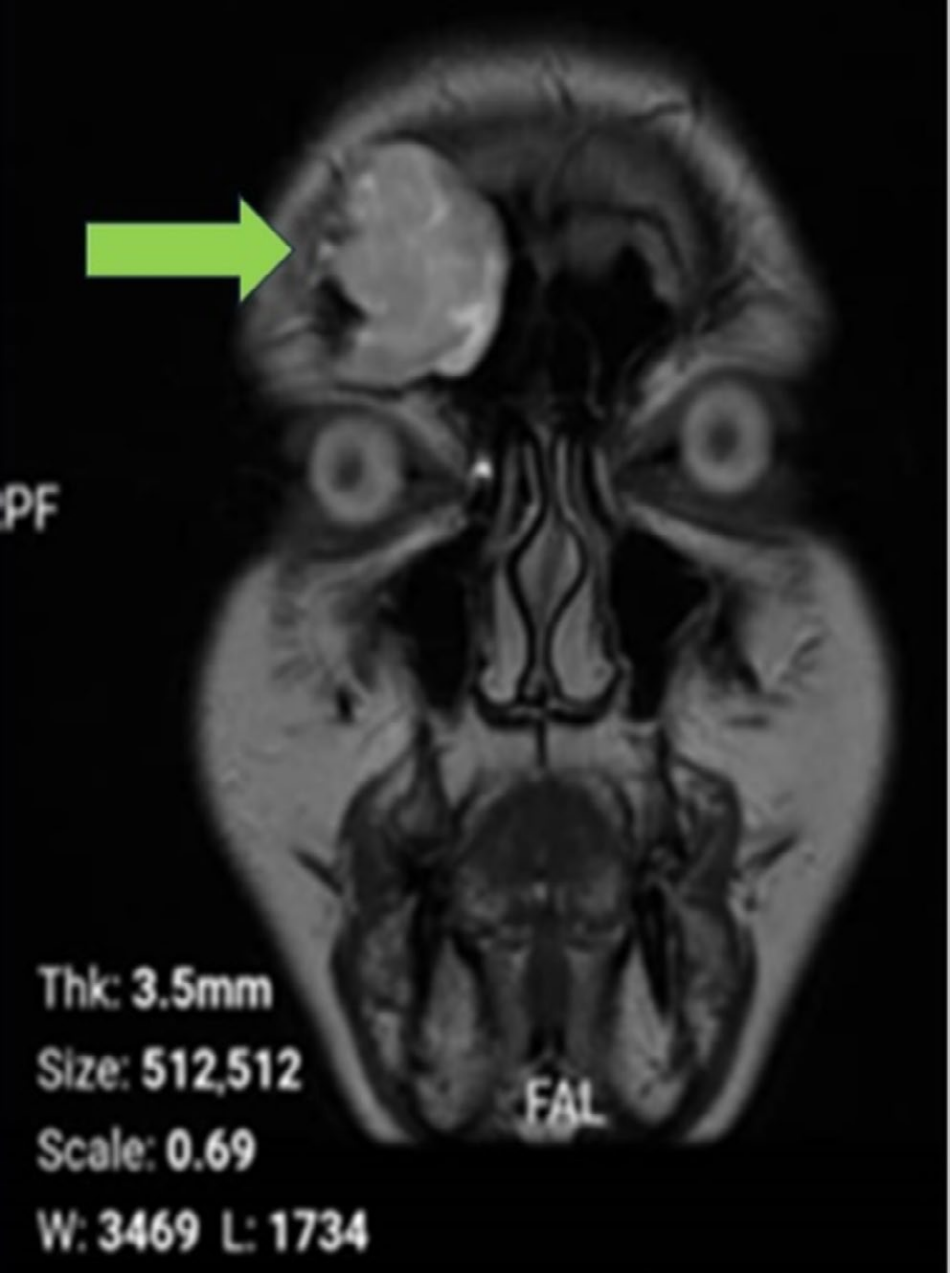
Coronal T2‐weighted MRI revealing a intermediate to mildly hyperintense lesion in the frontal sinus with inferior displacement of surrounding tissues.
